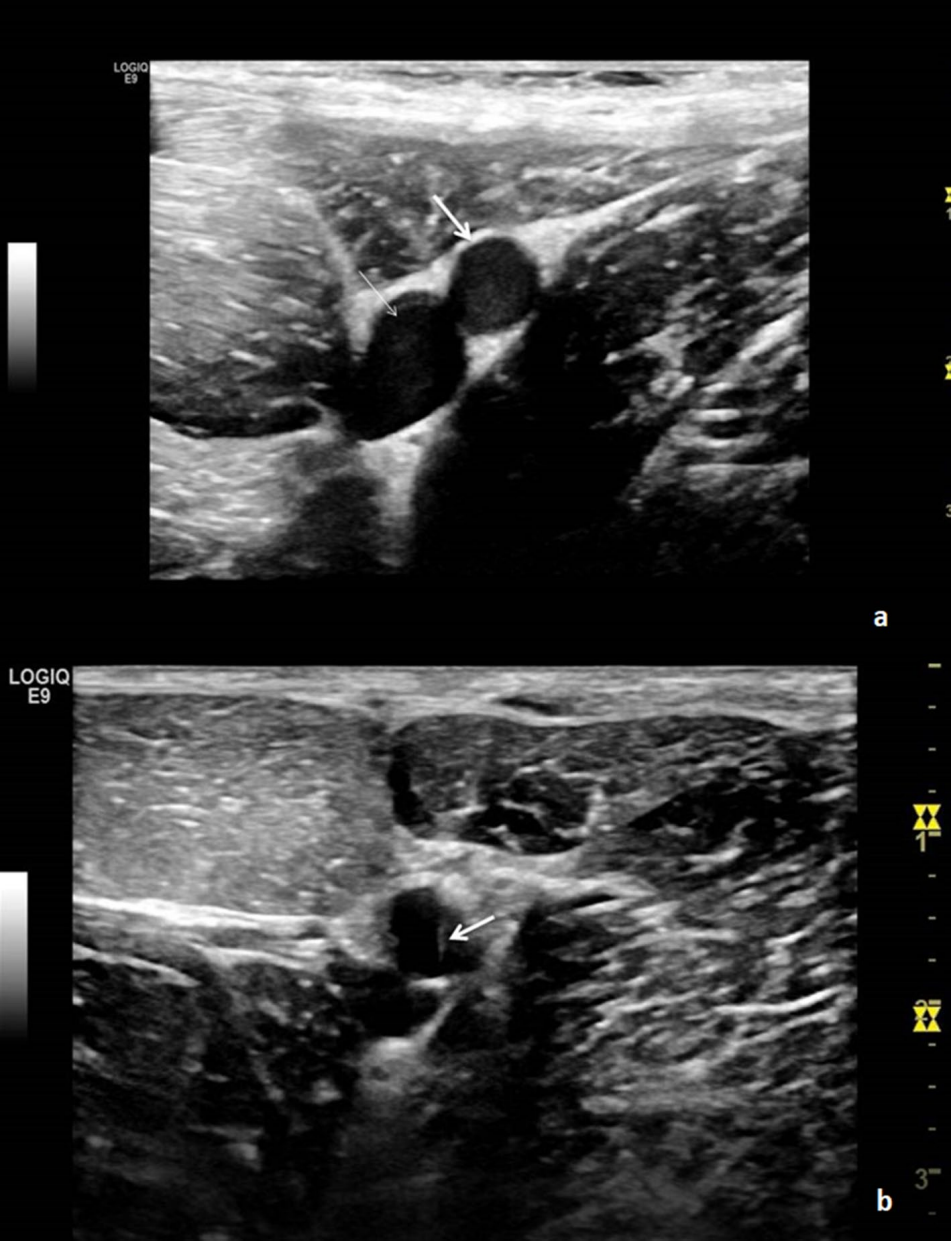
(a) Femoral artery (thick arrow) and vein (thin arrow), (b) ultrasound-guided puncture of the SFA. White arrow shows the puncture needle inserted into the SFA.
